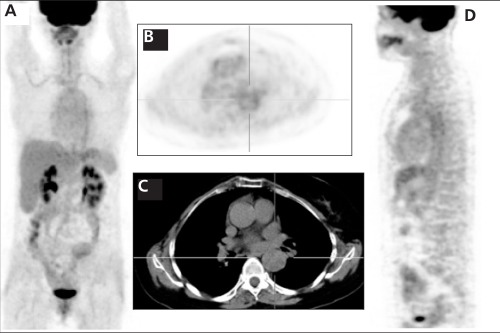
The same patients’ follow up F 18 FDG PET/CT images after2 months of immunosuppressive therapy. Figure 2A: MIP, 2B: AxialPET, 2D: Sagittal PET showed almost complete disappearance of largevessels’ F 18 FDG uptake but also minimal F 18 FDG uptake in theaorta and bilateral subclavian arteries. After therapy, the maximumstandardized uptake value of the aorta was 3.4. Figure 2C axial CTimages showed no pathological lesion or mass in the large vessels
